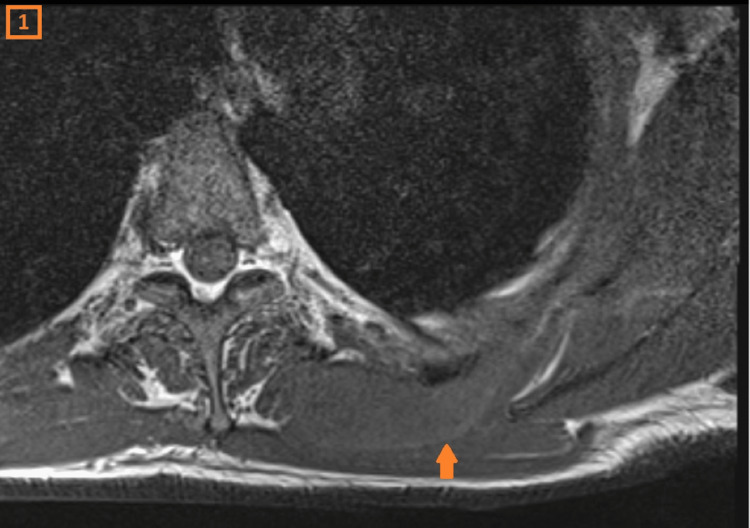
Cystic lesion as seen on axial T1-weighted image
